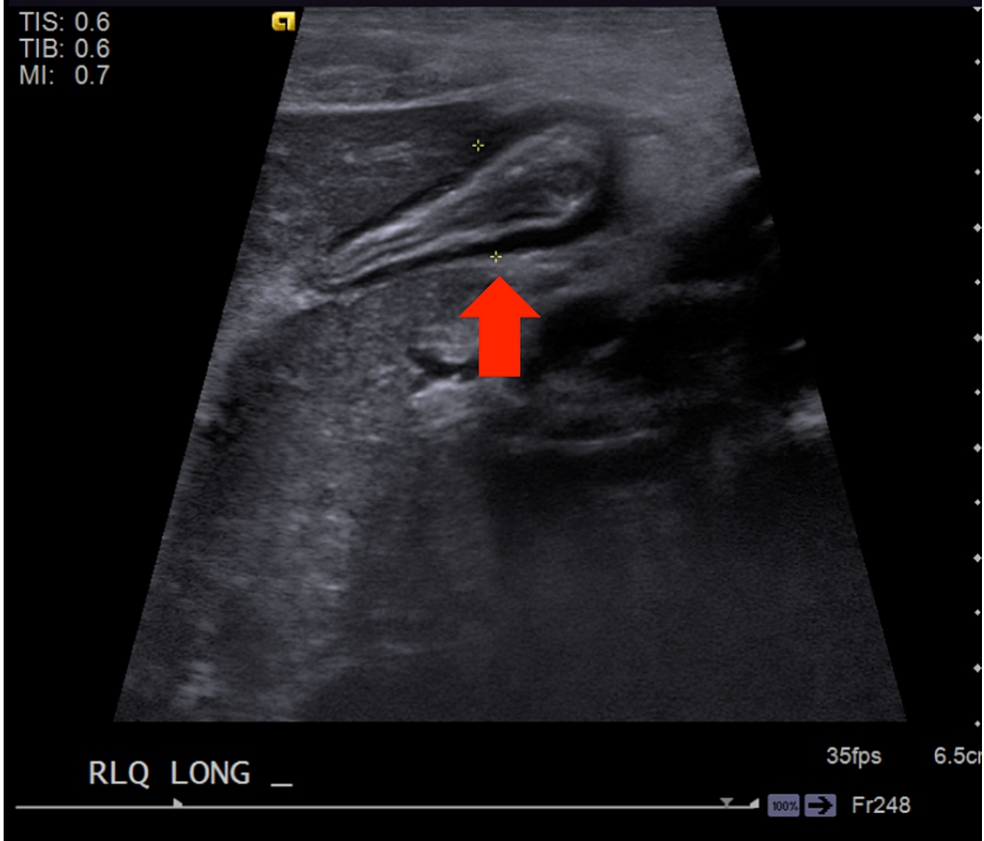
Sonographic examination of the right lower quadrant The appendix is identified within the right lower quadrant (red arrow). The finding is non-compressible and measures approximately 1 cm in diameter. Additionally, there is edema of the wall of this structure.
